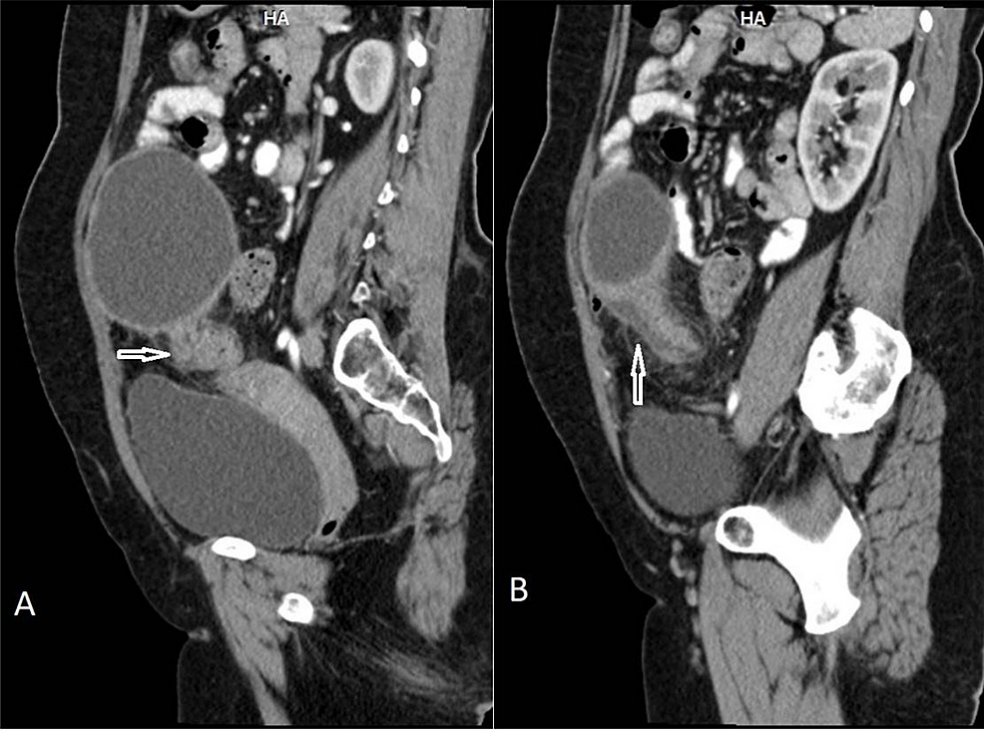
Case 2 CT sagittal reformations (A & B) showing ‘helical swirl’ appearance of twisted pedicle between the cystic lesion and the uterus (marked by arrows)
